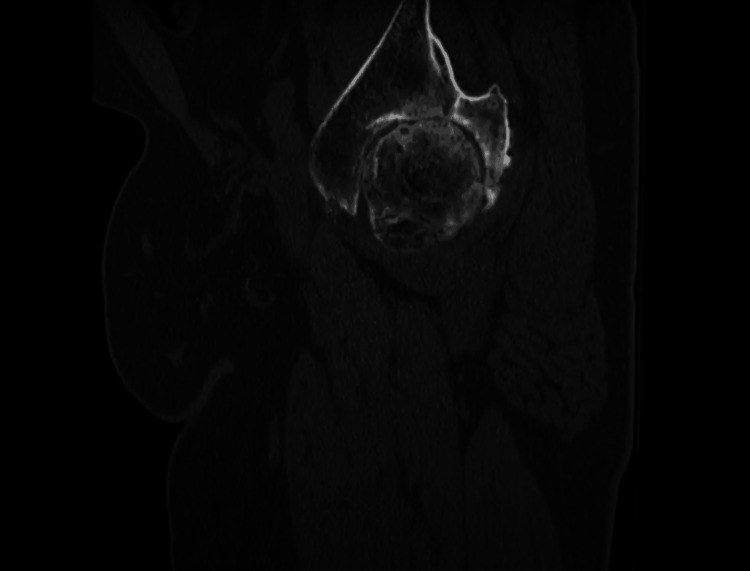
Sagittal CT scan of the left femoral acetabular joint.
